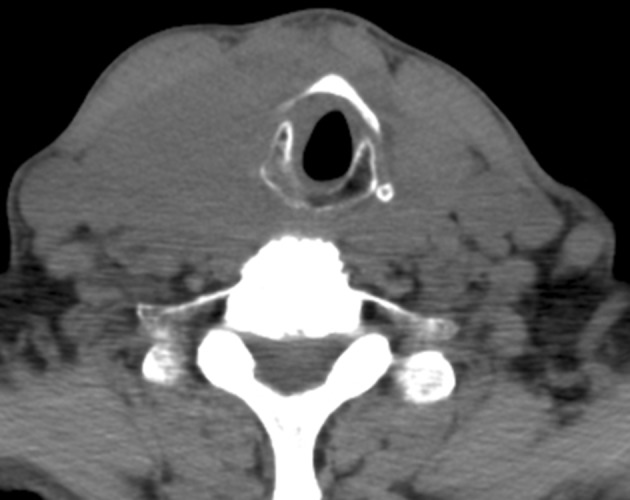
The CT Scan Showing Lack of Significant Tracheal Compression
